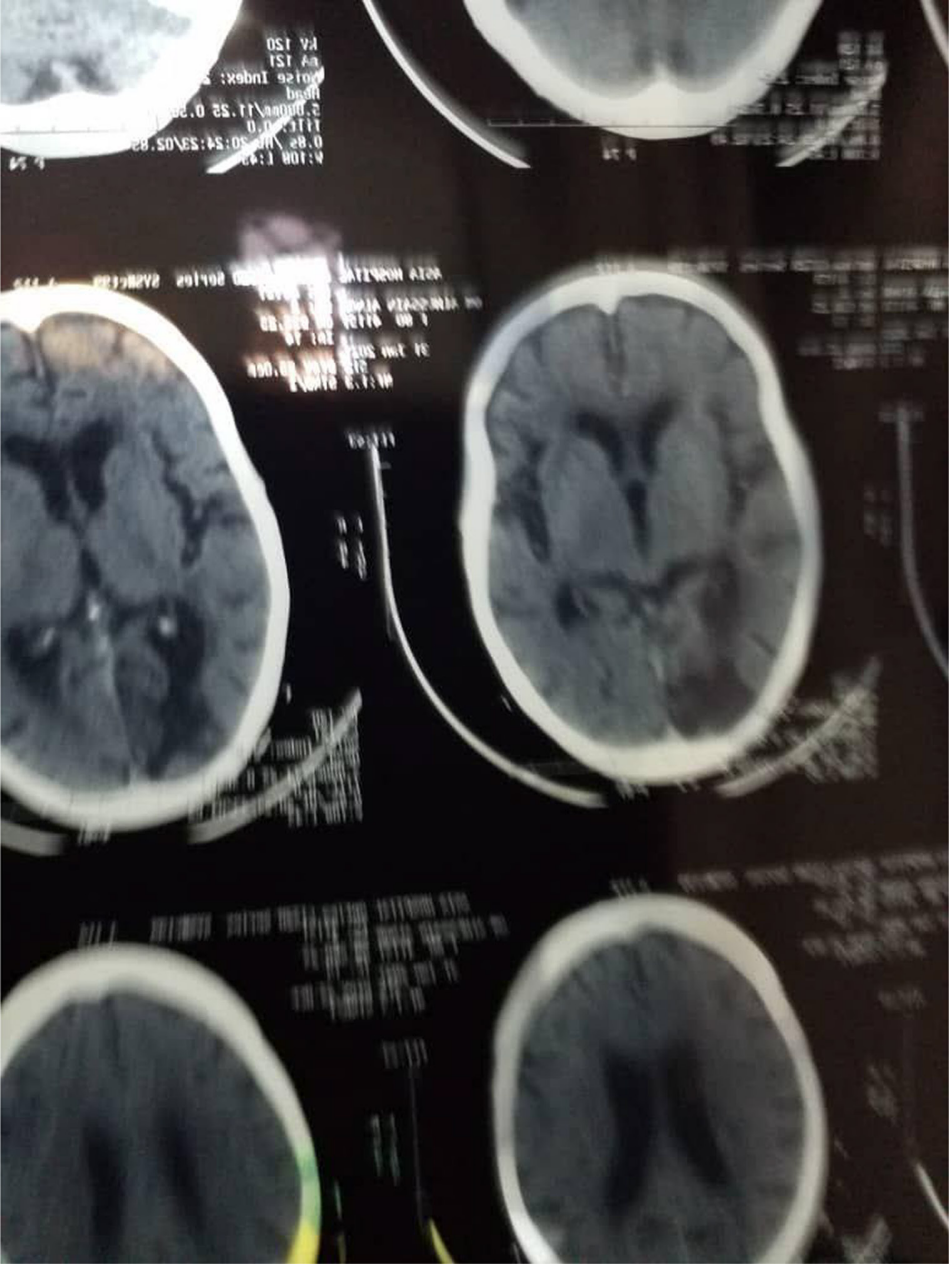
CT brain showed cerebral infarction
